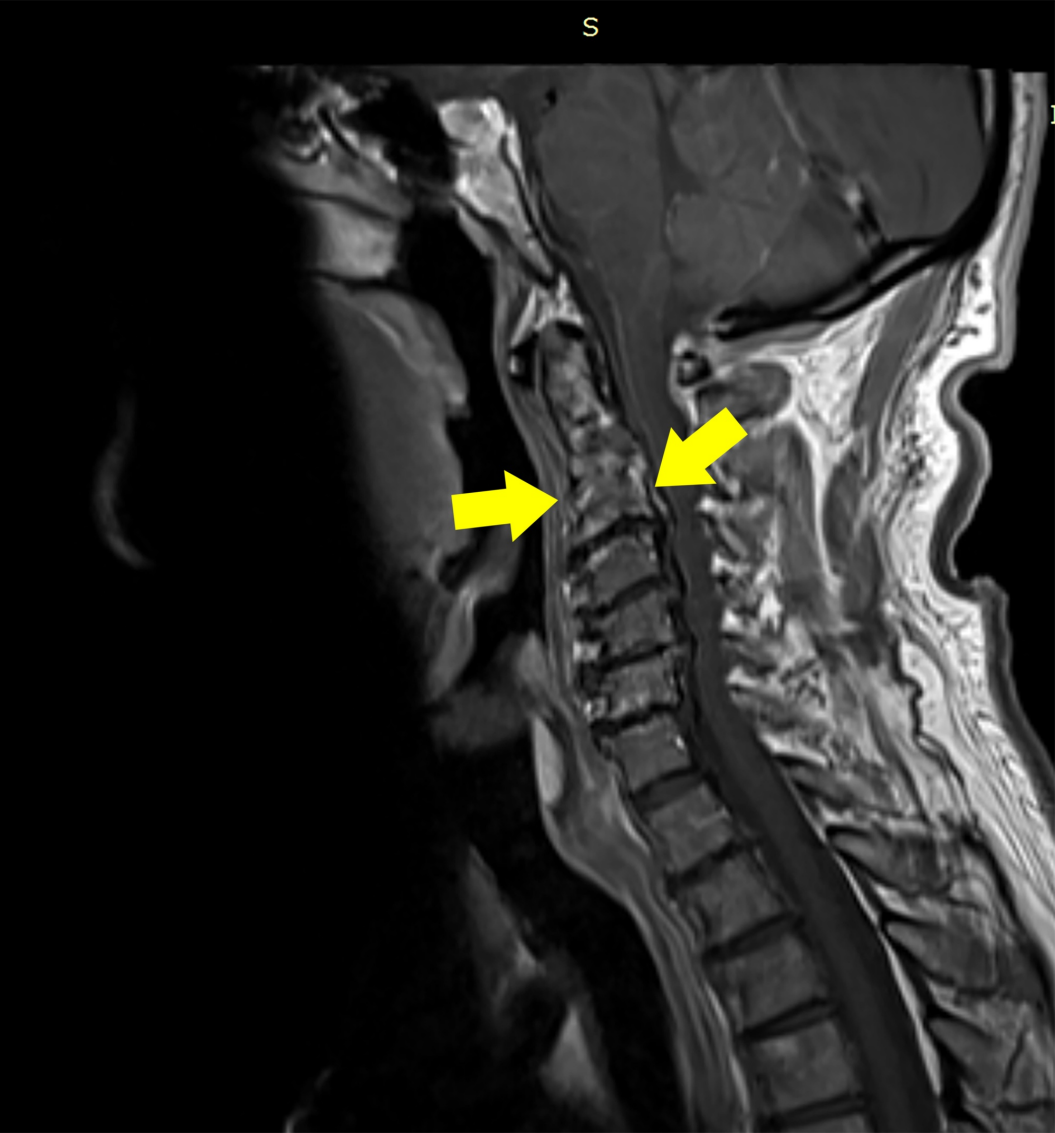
Follow-up MRI scan Four-month follow-up MRI showing resolution of retropharyngeal and epidural abscess (yellow arrows)
